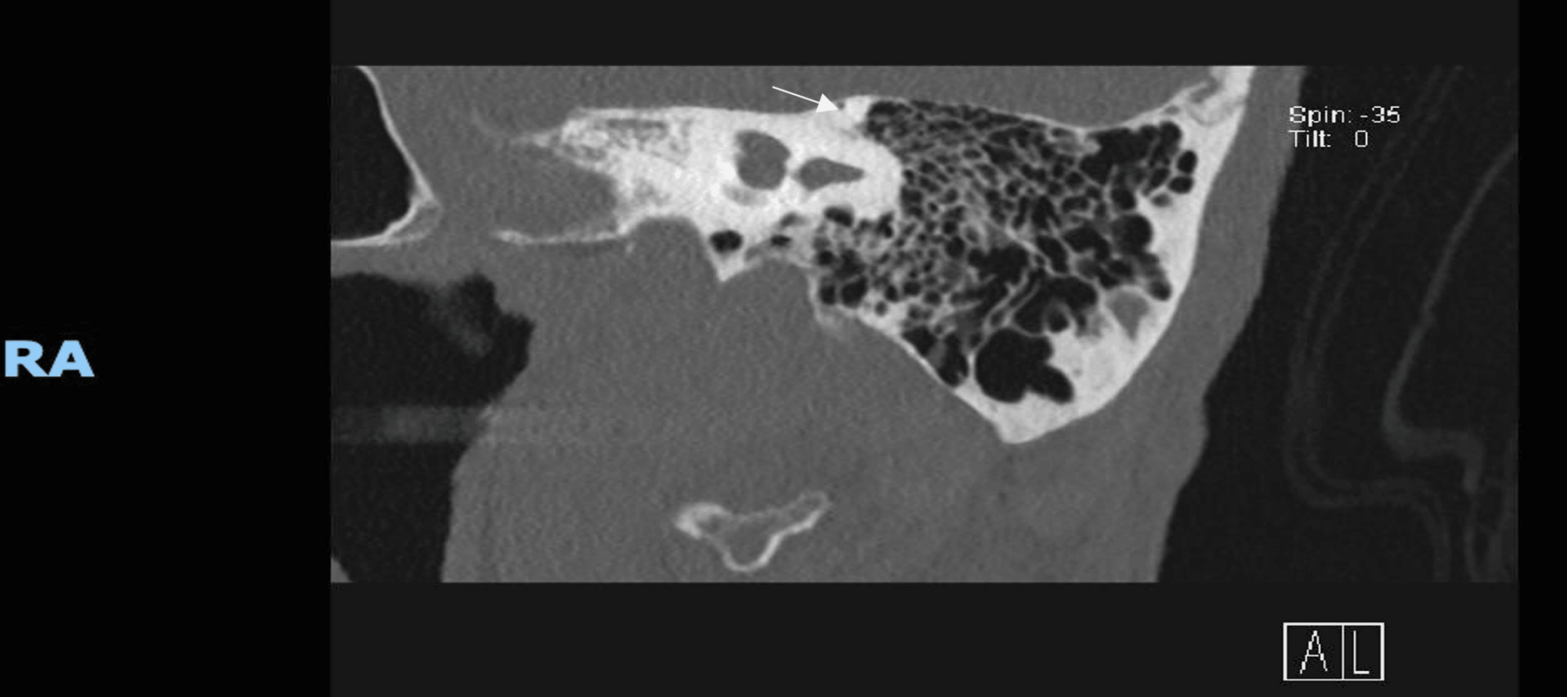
Coronal high-resolution CT of the left temporal bone revealing similar dehiscence of the superior semicircular canal, confirming bilateral involvement. Note the continuity of the membranous canal with the cranial cavity, suggestive of dual-sided "third window" physiology CT: computed tomography
